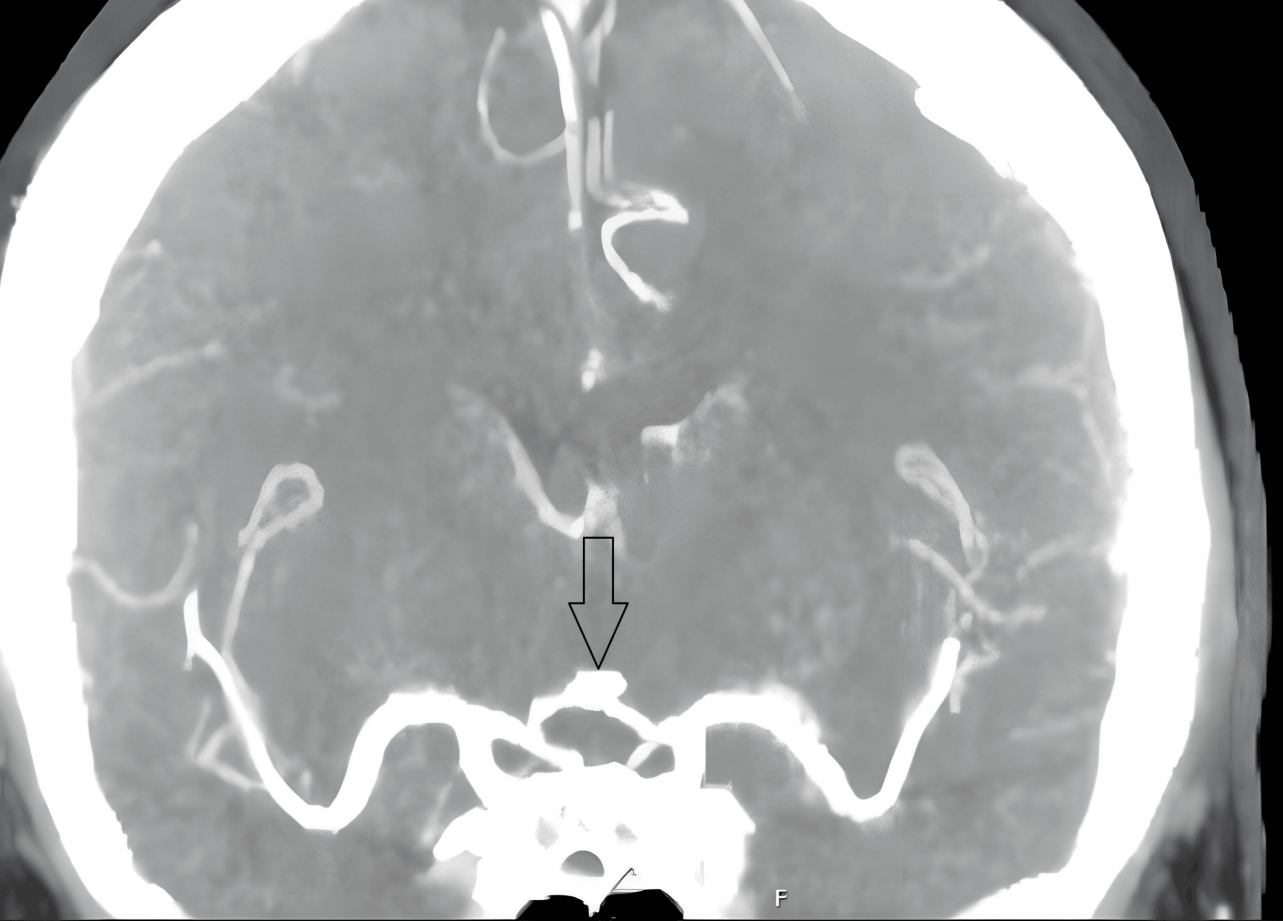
CTA Circle of Willis: 4 mm basilar tip aneurysm. Possible minor focal dilatation also seen in the left A2 segment measuring 1.7 mm (arrow). The intracranial segments of the internal carotid arteries and the major vessels forming the Circle of Willis and their branches are of normal calibre and are patent.
